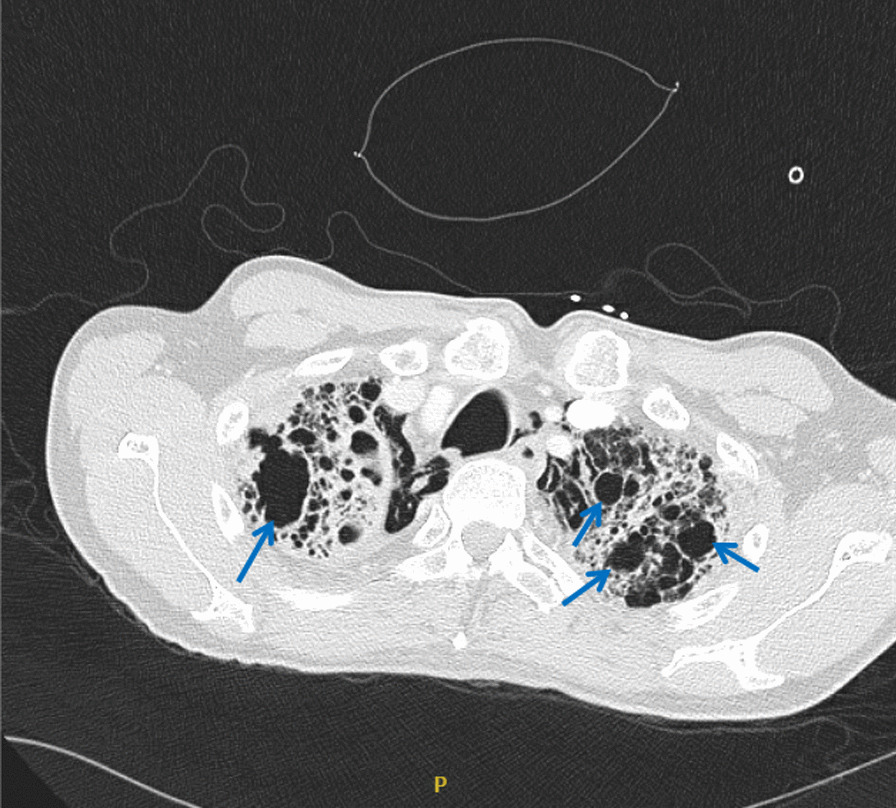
Cavitary lesions in a patient with *M. kumamotonensis* infection and underlying pulmonary fibrosis. Chest CT showed bilateral upper lobe cavitary lesions (see arrows) and honeycombing. Cavities in the right apex are more prominent than left apex
